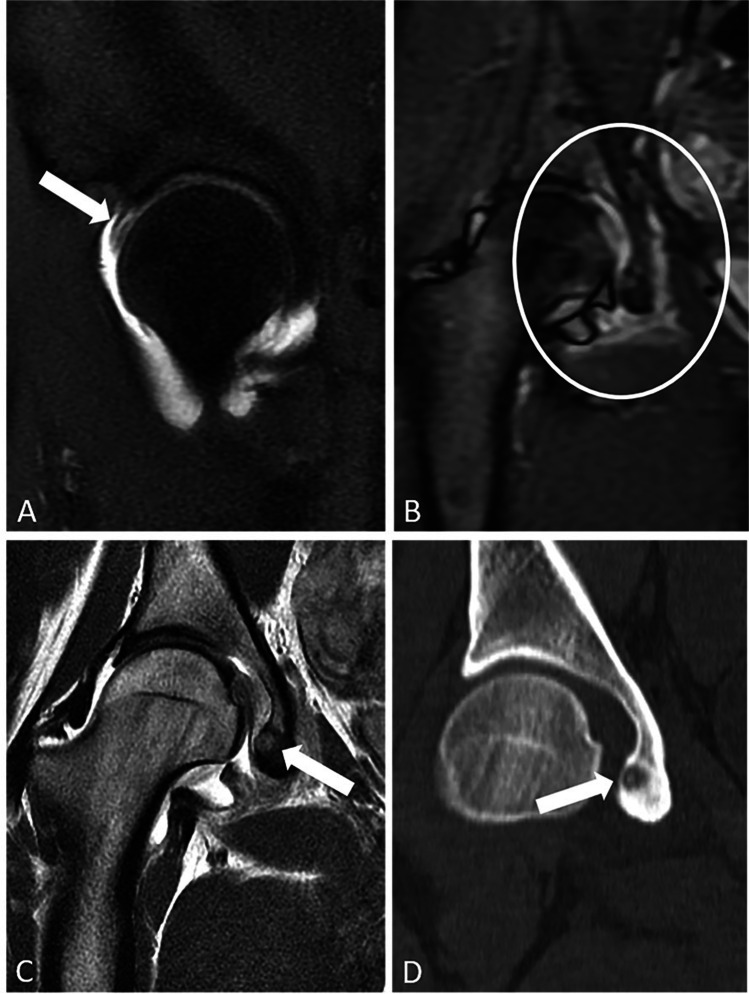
A 16-year-old female with a clinical diagnosis of FAI was referred for an MRI arthrogram to evaluate for a labral tear. Sagittal T1 weighted MRI (A) with intra-articular gadolinium shows an anterosuperior labral tear (arrow). Large field-of-view coronal STIR image of pelvis and hips (B) shows asymmetric increased T2 signal compatible with edema in the bone and soft tissues along the medial wall of the right acetabulum (circle), which would be unexpected with a diagnosis of FAI. Coronal PD image of the hip shows a tiny round lesion in the medial wall of the acetabulum near the epicenter of the edema (C), which was seen to better advantage and confirmed to represent an OO with marked surrounding sclerosis on coronal CT (D)
